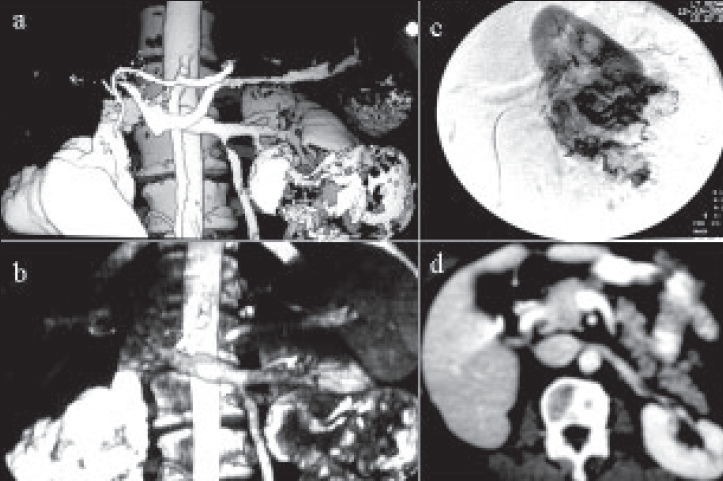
Spiral CT angiogram showing bilateral renal tumor. Right side radical nephrectomy and left lower polar partial nephrectomy was performed. a) and b) 3-D view, c) renal DSA of left side, d) postoperative follow-up CT scan after 3.8 years
